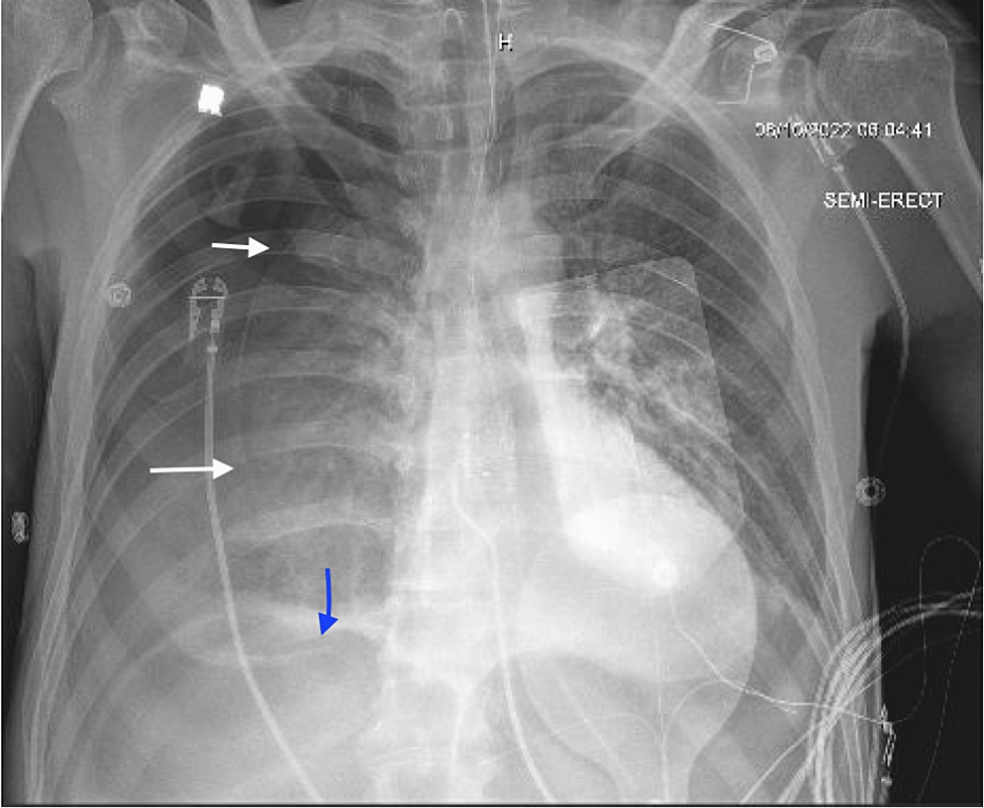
Chest X-ray showing pneumothorax (white arrows) and lucency under the diaphragm, suggestive of free intraperitoneal air (blue arrow).
